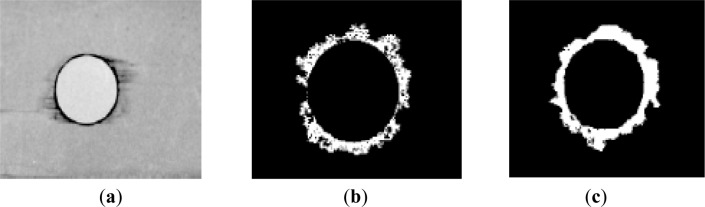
Damage evaluation: (a) radiography [26]; (b) ultrasonic C-Scan [28]; (c) computerized tomography [28].
